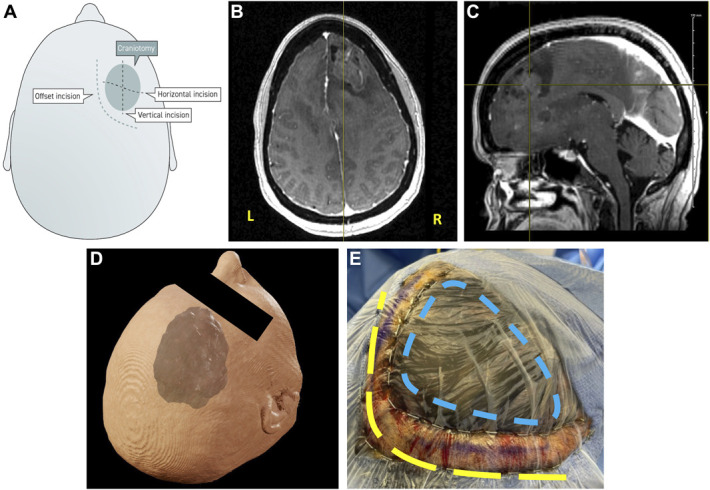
Offset incision design. A, Potential incisions for a given craniotomy. B, Axial and C, sagittal views of brain MRI used in surgical planning of a representative patient with a right frontal tumor. D, 3-dimensional rendering of patient's tumor, including nonenhancing tumor elements. E, Approach to surgical planning, showing the approximate needed craniotomy size (blue dashed line), offset scalp incision (purple line), and approximate stairstep pericranial incision (yellow dashed line).
